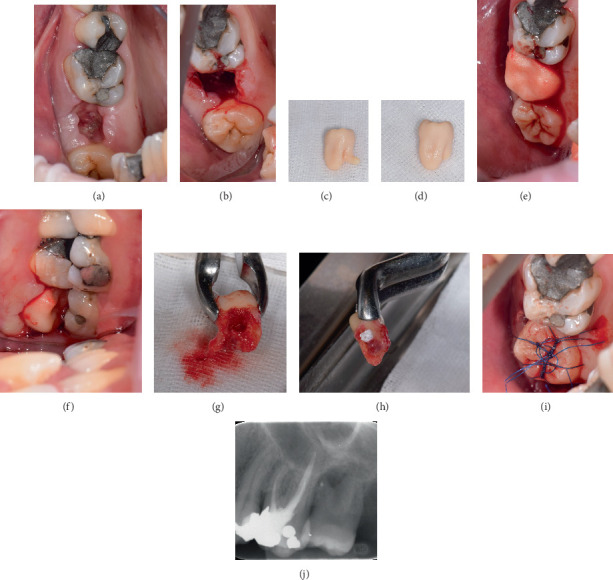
Surgical procedure. (a) The wound healing 2 weeks after the extraction prior to surgery. (b) The preparation of the recipient socket just by excision of granulation tissue. (c, d) The CARP model was adjusted according to the assumed fracture line, and (e, f) the position inside the socket was checked. (g) The donor tooth was atraumatically extracted, utilizing forceps only, and the buccal root remained intact and was removed using a diamond bur in a slow handpiece. (h) The root canal was shaped using an ultrasonic diamond-coated instrument and filled with MTA. A suspected area 0.8 × 1 mm devoid of PDL at the opposite site was visible. (i) The tooth was placed in the recipient socket and secured by suture. (j) Immediate postoperative periapical radiograph.
